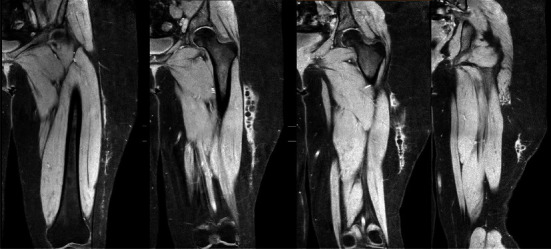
MRI T2 sequence of the left thigh approximately 13 months post-injury demonstrating decreased size of the lateral fluid collection superficial to the deep fascia of the left thigh.
